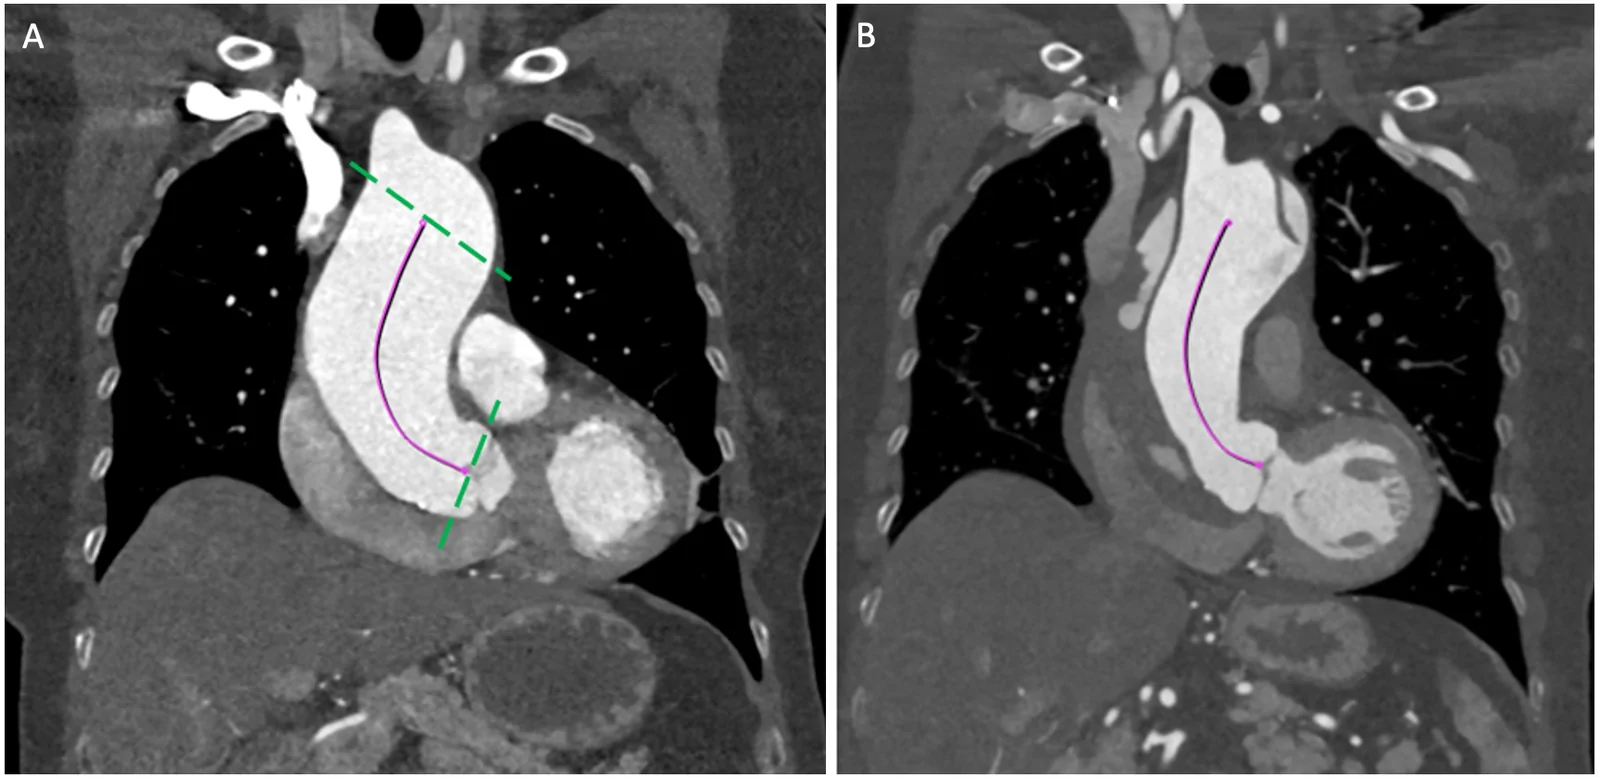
The AAL automatic measurements in multislice computed tomography angiography (CTA) were performed along the software-generated central line (purple) with syngo.via (siemens AG, Germany). Panel A. The AAL is measured with syngo.via from the aortic annular plane (proximal dotted green line) to the origin of the brachiocephalic artery (distal dotted green line). Prior to acute aortic dissection AAL was equal to 97.8 mm. Panel B. The AAL after acute aortic dissection incremented to 98.4 mm. AAL: ascending aortic length.
